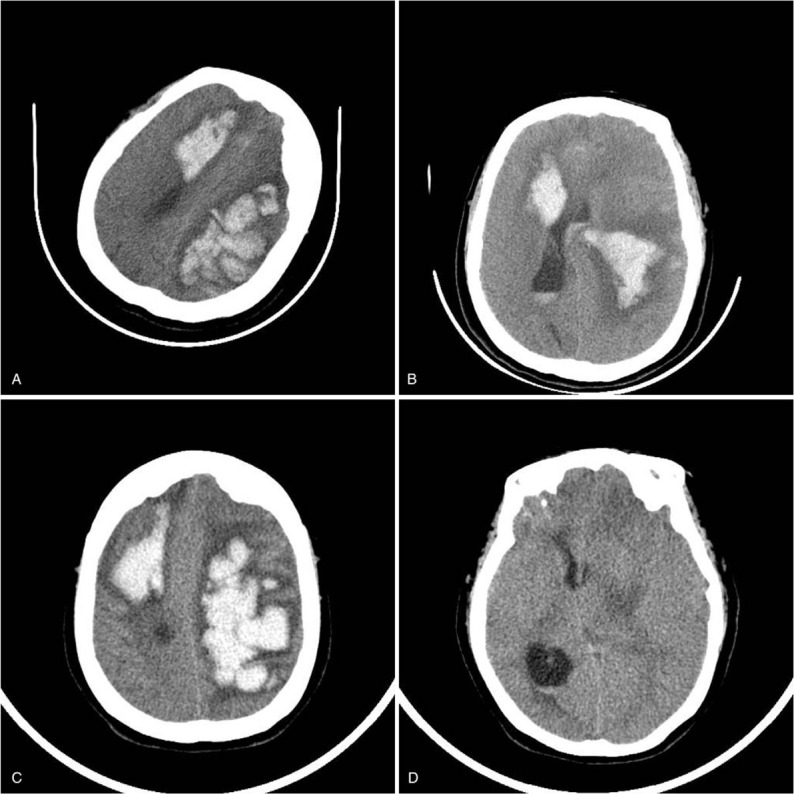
Multiple head CTs. (A) Multiple lobar hemorrhages (October 5th); (B) cerebral hemorrhage of bilateral frontal lobe and left parietal lobe breaking into the ventricle (October 6th); (C) bilateral cerebral hemisphere hemorrhage breaking into the ventricle and subarachnoid space with cerebral hernia (October 12th); (D) slight high density irregular shadow in the right frontal and left parietal lobes and edema in the surrounding brain parenchyma show that the ventricle was obviously under pressure and the brain midline shifted right (October 12th). CT = computed tomography.
